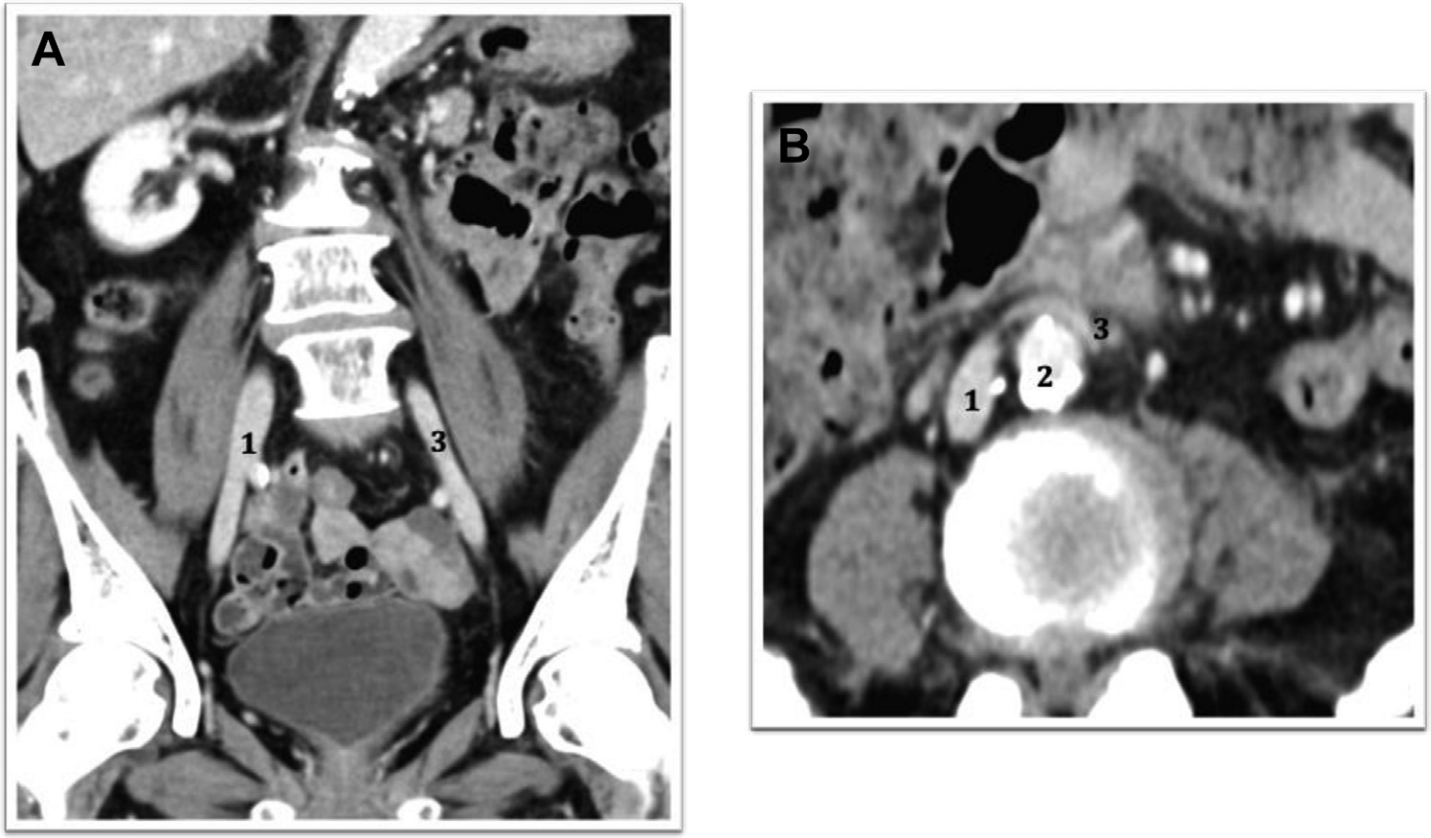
A, Coronal view of contrast-enhanced computed tomography scan 3 month after surgery showing a right inferior vena cava (IVC; *1*) and a patent left IVC (*3*). B, Axial view of contrast-enhanced computed tomography scan 3 month after surgery showing a patent left IVC (*3*) extending over the abdominal aorta (*2*) to the right IVC (*1*).
